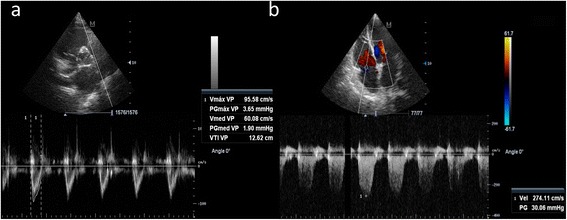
Example for estimating the pulmonary vascular resistance. a. Right ventricle outflow tract velocity time integral (12.6 cm) obtained in parasternal short-axis view. b. Right ventricle to right atrial peak velocity (2.7 m/s) obtained from a tricuspid regurgitation jet in apical four-chamber view. PVR is obtained as (2.7 /12.6) × 10 + 0.16, equal to 2.3 Wood units (normal <1.5)
